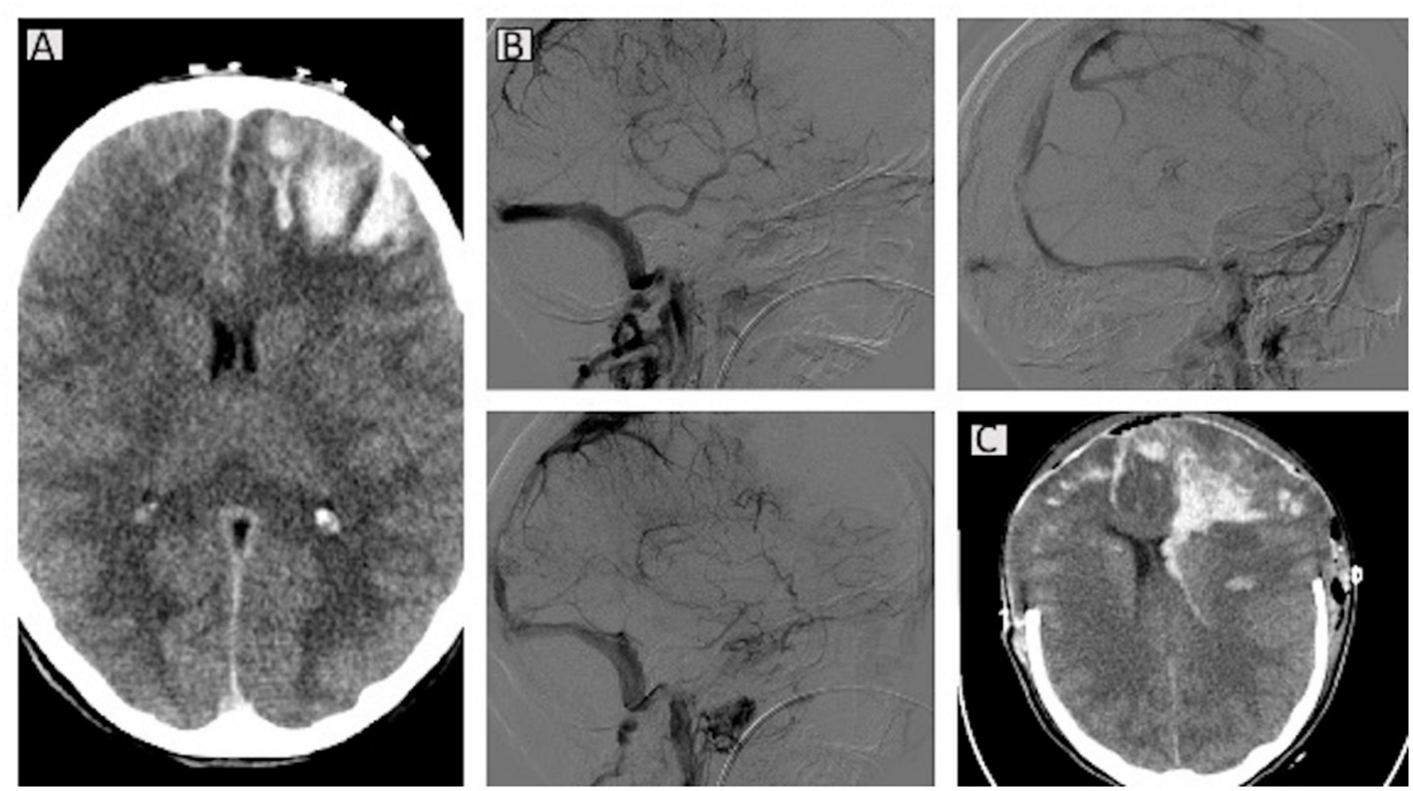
Computed tomography (CT) scans for case 2. A brain CT scan showed bilateral venous infarct with hematic component in the fronto-basal-anterior and parietal areas and associated intraventricular hemorrhage. Initial cerebral herniation of the cerebellar tonsils is present (A). At angio-CT, the arterial circulation is patent, whereas the superior sagittal sinus appears obliterated, the sinus recto is thinner, and the cavernous sinus is partially matted (B). Bilateral decompressive craniectomy and midline shift are evident in the latest CT scan (C).
